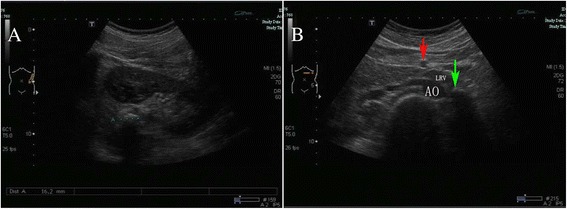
The images from the ultrasonography. a An abdominal ultrasonography demonstrated 16-mm hyperechoic, echogenic focus in the left renal pelvis. b A color Doppler ultrasonography indicated the hyperechoic focus (*green arrow*) in the left renal vein (LRV). Aorta (AO); left renal vein (LRV); superior mesenteric artery (*red arrow*)
